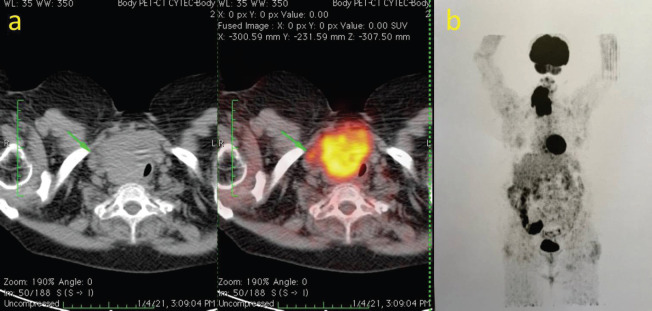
(a): CT scan and ^18^.FDG.PET.CT scan, at initial presentation (axial). (b): ^18^.FDG.PET scan, coronal view.
